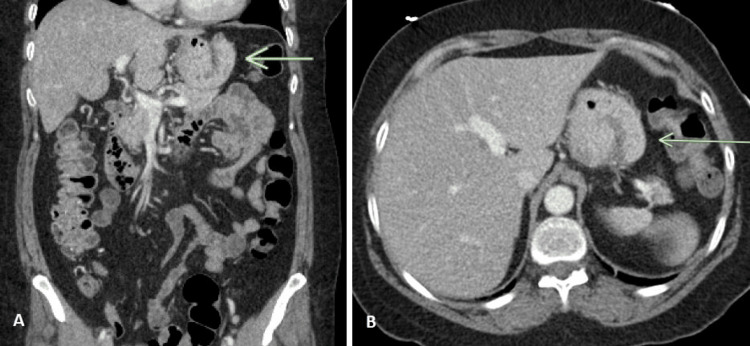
CT of the abdomen and pelvis demonstrating folding of the gastric cardia onto itself on both coronal (A) and axial (B) views (arrows) CT: computed tomography
